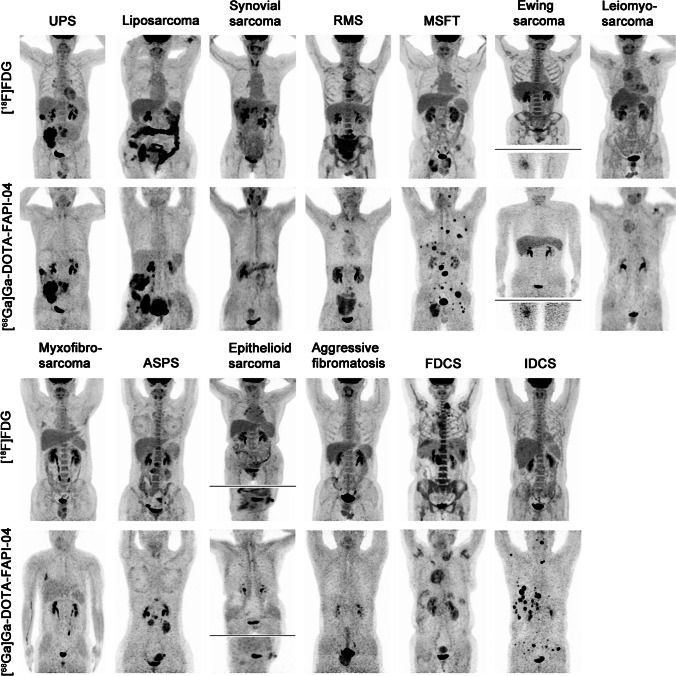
MIP images of [^18^F]FDG PET/CT and [^68^ Ga]Ga-DOTA-FAPI-04 PET/CT in patients reflecting 13 different representative recurrent STS entities. MIP, maximum-intensity projection; STS, soft tissue sarcoma; UPS, undifferentiated pleomorphic sarcoma; RMS, rhabdomyosarcoma; MSFT, malignant solitary fibrous tumor; ASPS, alveolar soft part sarcoma; FDCS, follicular dendritic cell sarcoma; IDCS, interdigitating dendritic cell sarcoma
